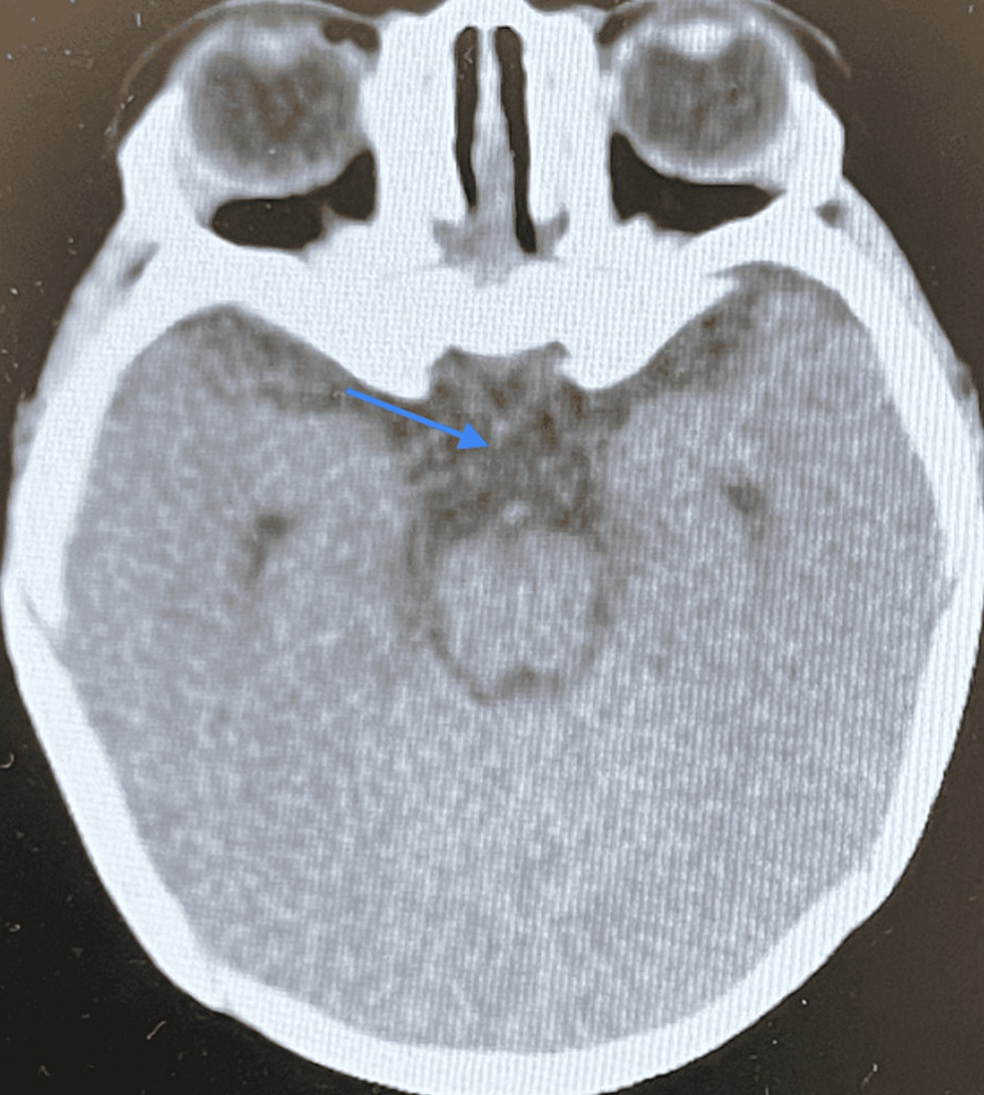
Non-contrast CT brain scan: posterior fossa structures degraded.
